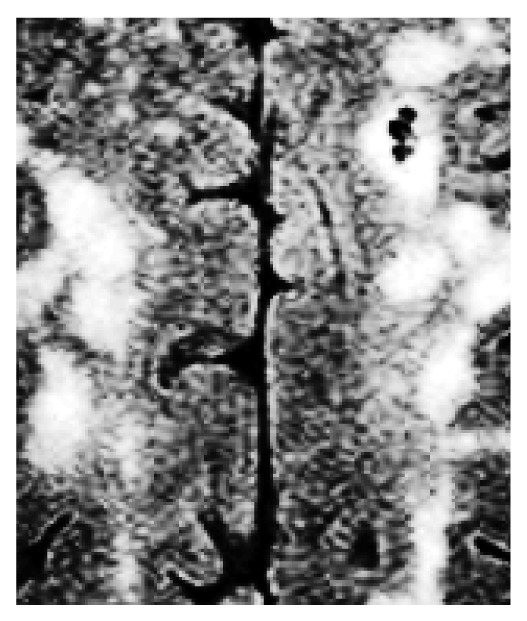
Enhancement of the brain MRI using histogram equalization.
